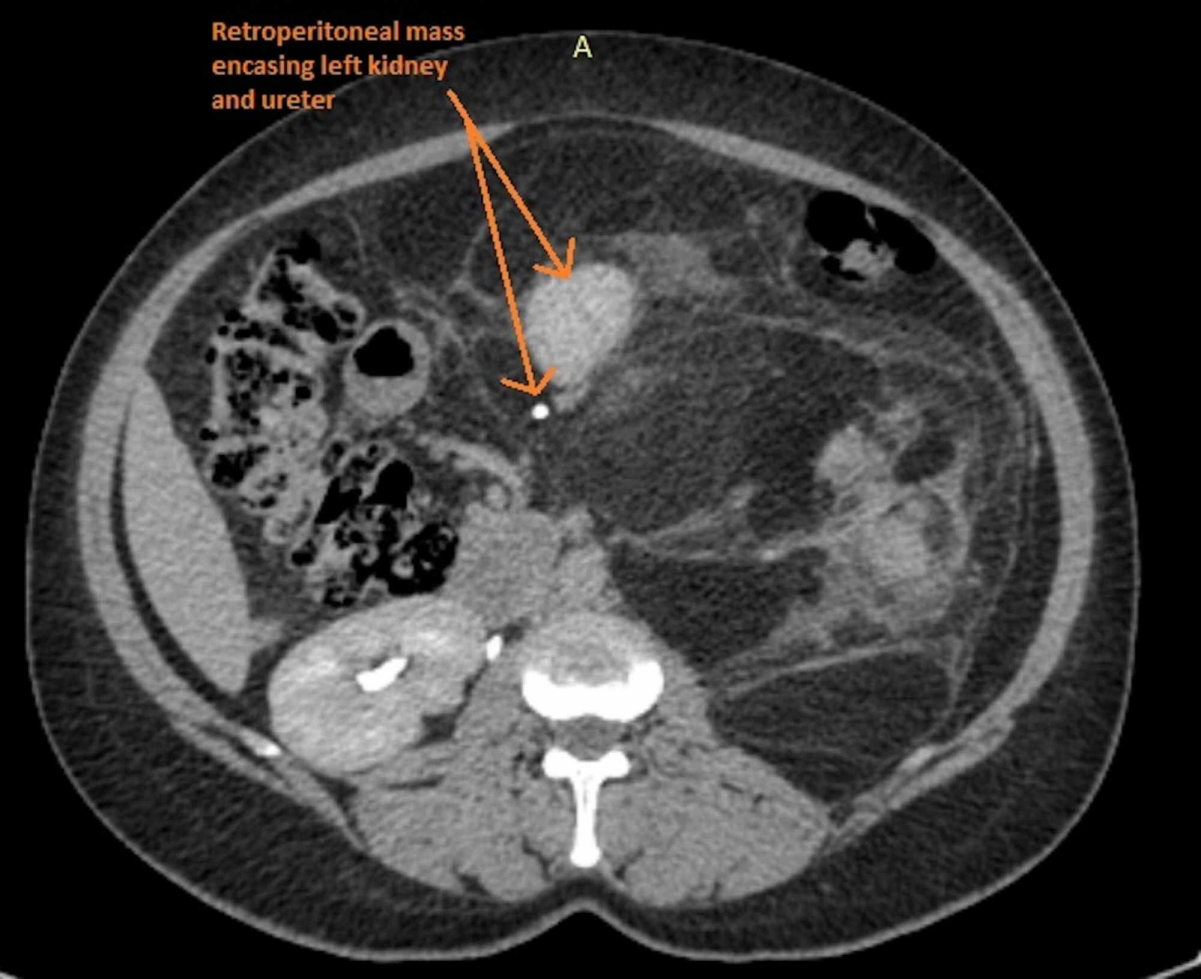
An intravenous contrast CT image showing complete encasement of her left kidney, ureter, and the descending colon by the tumour (arrows).
